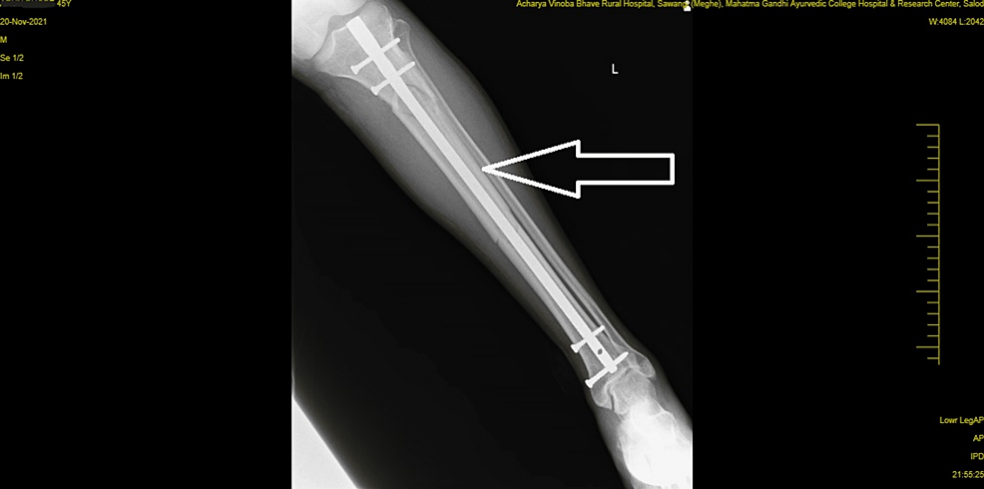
Postoperative (PA view) x-ray of the tibia showing interlocking. PA: posteroanterior.
